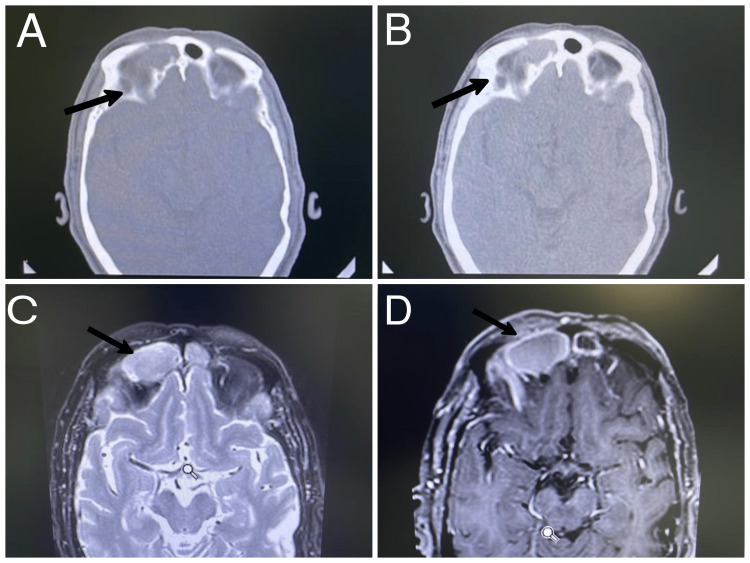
Case 1. Right frontal mucocele in male patient presenting with right eye ptosis, diplopia, and headache. (A) Axial CT scan bone window at level of the frontal sinus, showing expansile mass in right frontal sinus with bone thinning in both anterior and posterior table; (B) Soft tissue window; (C) T1 weighted MRI with gadolinium axial cut showing low signal with peripheral enhancement intensity; (D) T2 FLAIR MRI indicating high signal intensity lesion. FLAIR: fluid-attenuated inversion recovery
